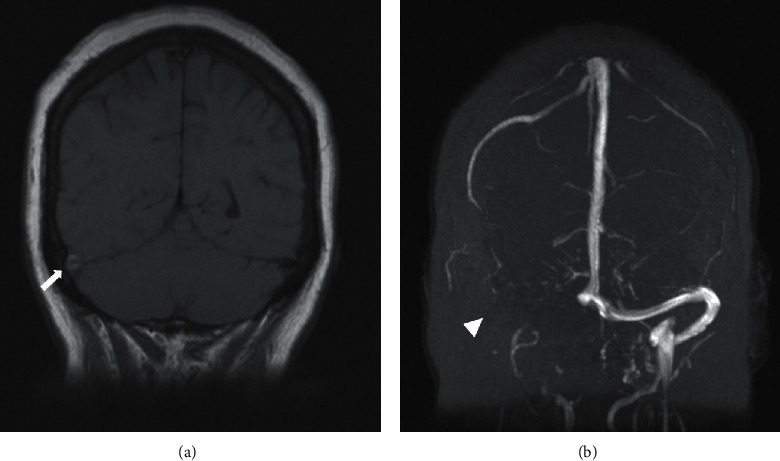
Magnetic resonance imaging demonstrating flow void at right transverse sinus (arrow) and absence of contrast (arrowhead) along transverse sinus consistent with transverse sinus thrombus.
